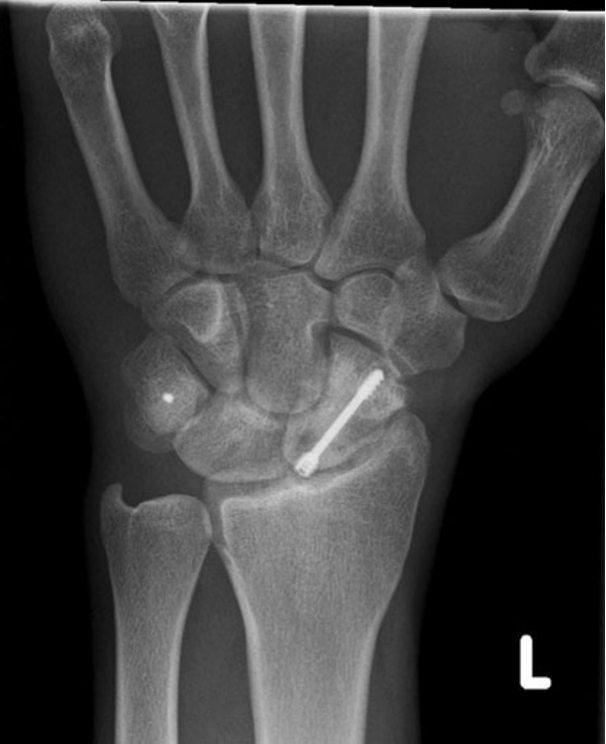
Wrist PA X-ray at 20 months suggesting scaphoid fracture union
